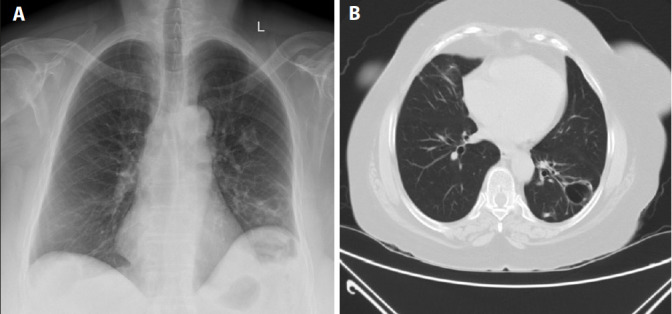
Repeat chest radiograph (A) and computed tomography images 6 months after initiation of therapy (B).
